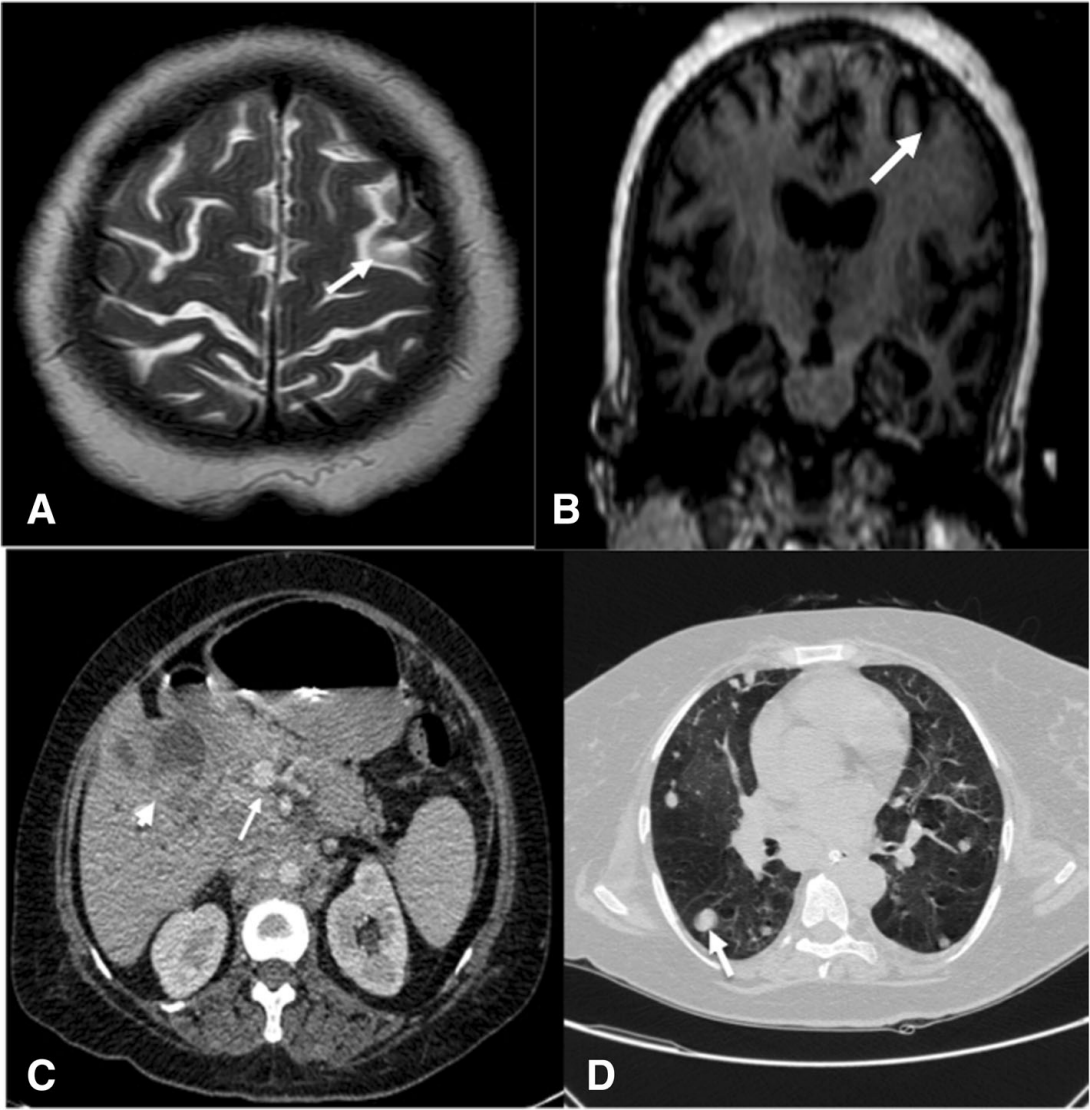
a MRI brain T2 weighted axial image showing encephalomalacia left frontal area with a corroborating signal intensity on T1 weighted coronal image (white arrow). b, c Contrast enhanced CT scan abdomen showing heterogeneously enhancing mass lesion in gall bladder (white arrow head) and enlarged periportal lymph node (white arrow). d CT thorax showing multiple metastatic lesion in lung parenchyma
